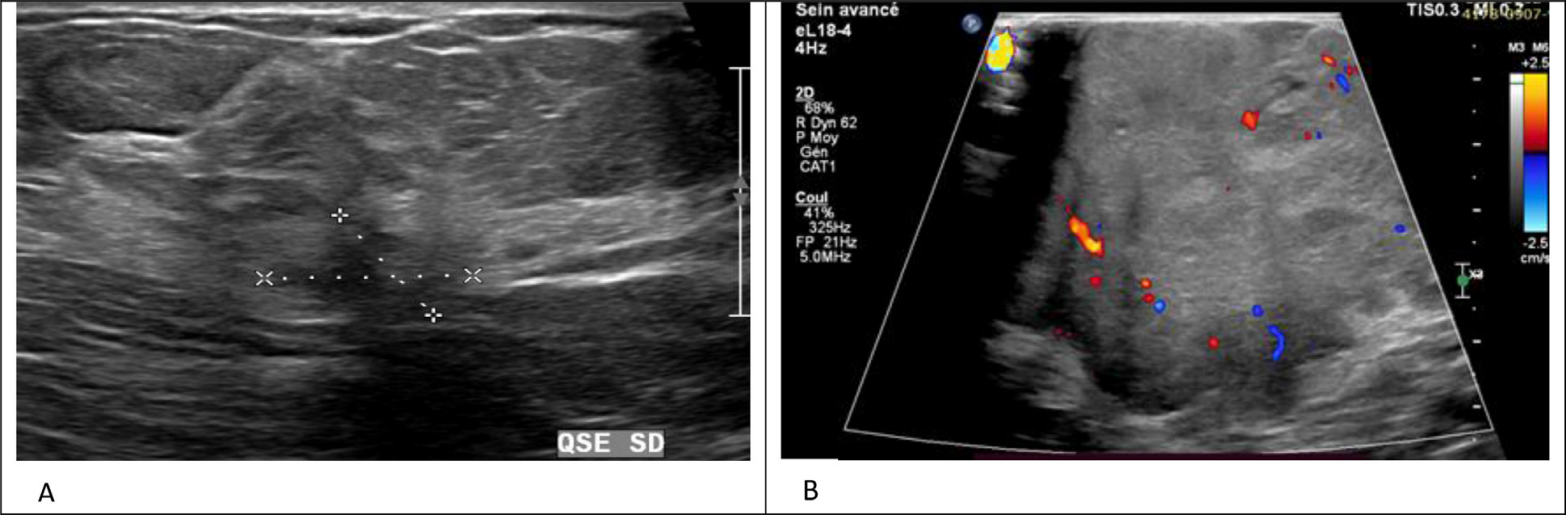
Ultrasonography of both breasts shows a spiculated, poorly circumscribed, hypoechoic mass with a posterior acoustic shadow in the left breast and an oval, irregular, heterogeneous mass with a diameter of 5 cm and rim vascularity in the left breast.
